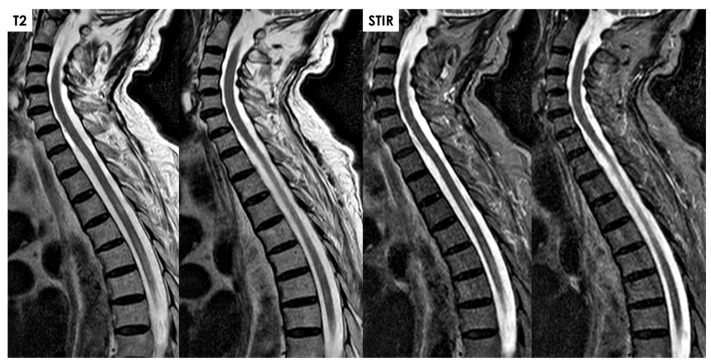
Same patient as in Figure 6. On the MRI performed three days after the onset, multifocal intramedullary lesions are evident, with extravasation at the level of the dorsal spinal cord. The first lesion spans from D3 to D4 for nearly two vertebral bodies, and the second spans D5-D6-D7 for at least three vertebral bodies, showing hyperintensity in T2 and STIR sequences, consistent with ischemic lesions. Axial scans and DWI are compromised by motion and respiratory artifacts.
